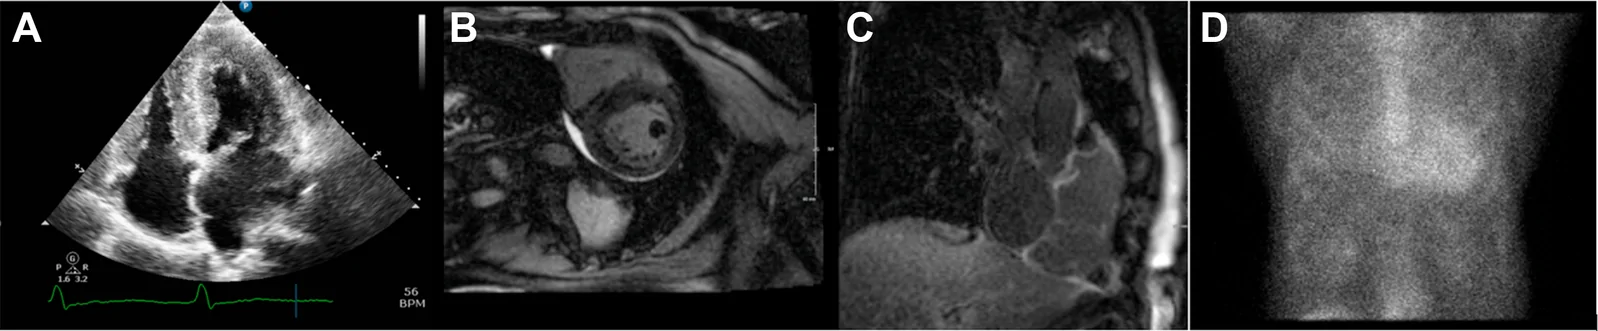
Multimodal Imaging: Echocardiogram, Cardiac Magnetic Resonance Imaging (cMRI) and 99mTc-Pyrophosphate (PYP) Scan (A) Apical 4-chamber view on echocardiogram showing biatrial enlargement, severe concentric left ventricular (LV) hypertrophy and increased LV wall thickness with speckled pattern. (B) Short-axis view on cMRI showing diffuse myocardial wall thickening with increased signal intensity. (C) Sagittal view on cMRI showing extensive late gadolinium enhancement. (D) PYP scan showing significantly increased uptake throughout the left ventricular myocardium, greater than bone marrow activity.
